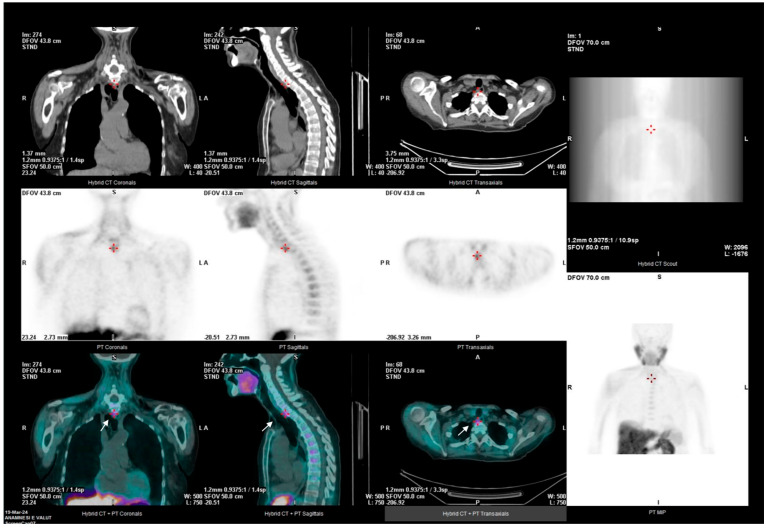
A 74-year-old woman diagnosed with normocalcemic primary hyperparathyroidism with bone complications. The 18F-fluorocholine PET/CT scan shows a posterior parathyroid, though it does not provide precise details regarding the origin of the localized gland.
